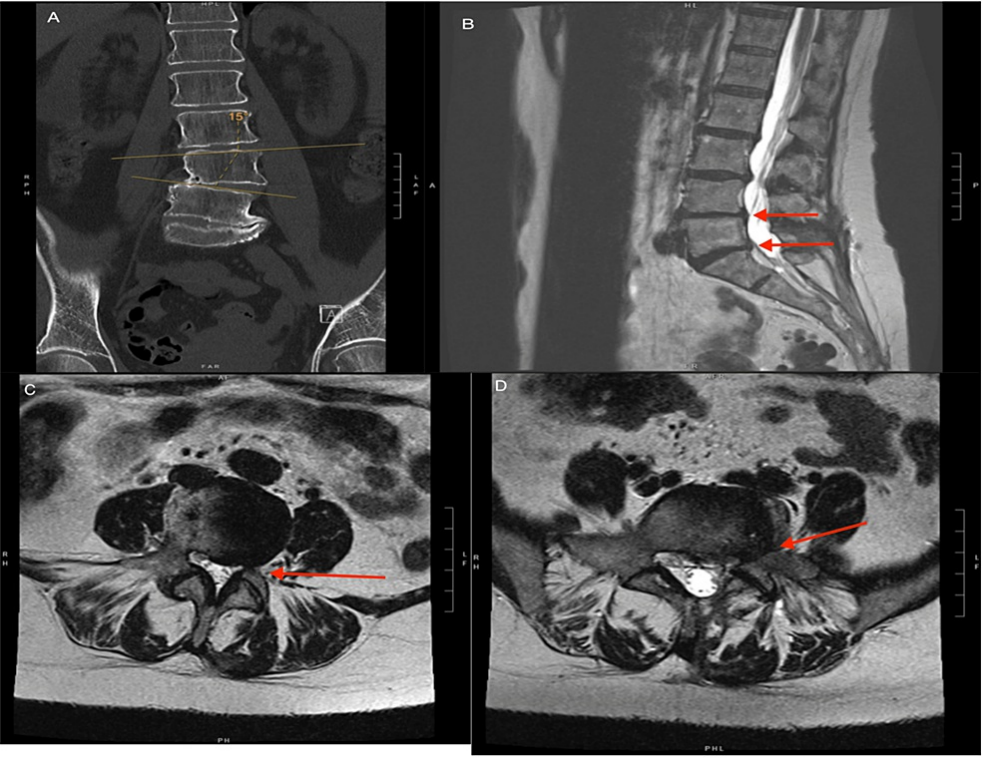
Preoperative Imaging: (A) coronal lumbar spine CT showcasing levoscoliosis measuring 15 degrees at L4-L5 with lateral listhesis to the left; (B) sagittal lumbar spine MRI showcasing L4/L5/S1 collapsed disc (red arrows) with degenerative disc disease and loss of disc height; (C) axial lumbar spine MRI showcasing L4/L5 left foraminal stenosis (red arrow); (D) axial lumbar spine MRI showcasing severe L5/S1 foraminal stenosis (red arrow). CT, computed tomography; MRI, magnetic resonance imaging
